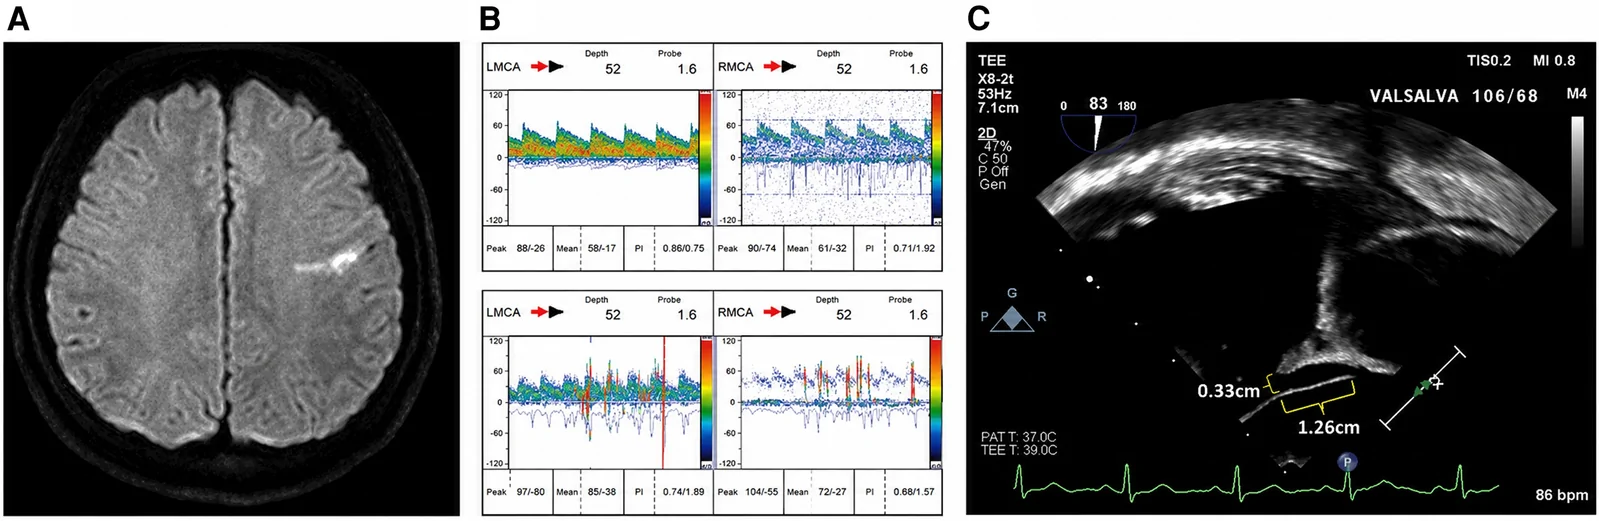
Diagnostic imaging. (*A*) Brain MRI demonstrating an acute infarction in the left precentral gyrus. (*B*) Transcranial Doppler bubble study with provocation demonstrating numerous high-intensity transient signals, consistent with a large right-to-left shunt. (*C*) Transoesophageal echocardiography demonstrating a long-tunnel patent foramen ovale.
